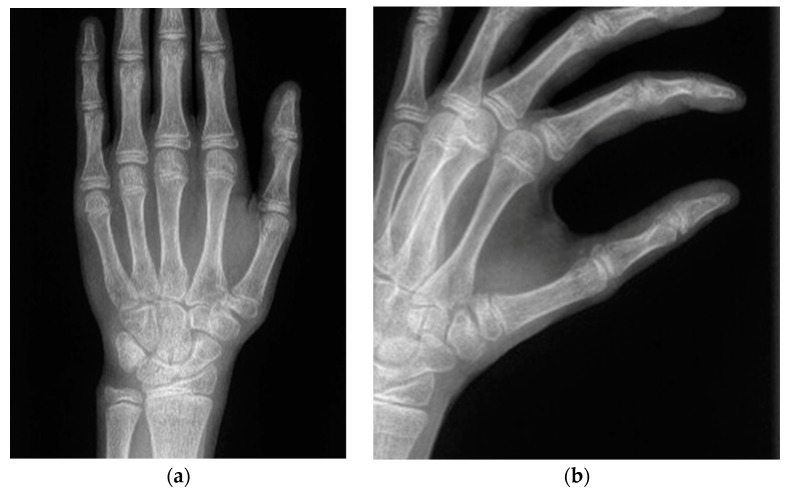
Plain radiographs of the TMC joint. (a) Roberts projection—strict AP view of the TMC joint. (b) Lateral view of the TMC joint.
